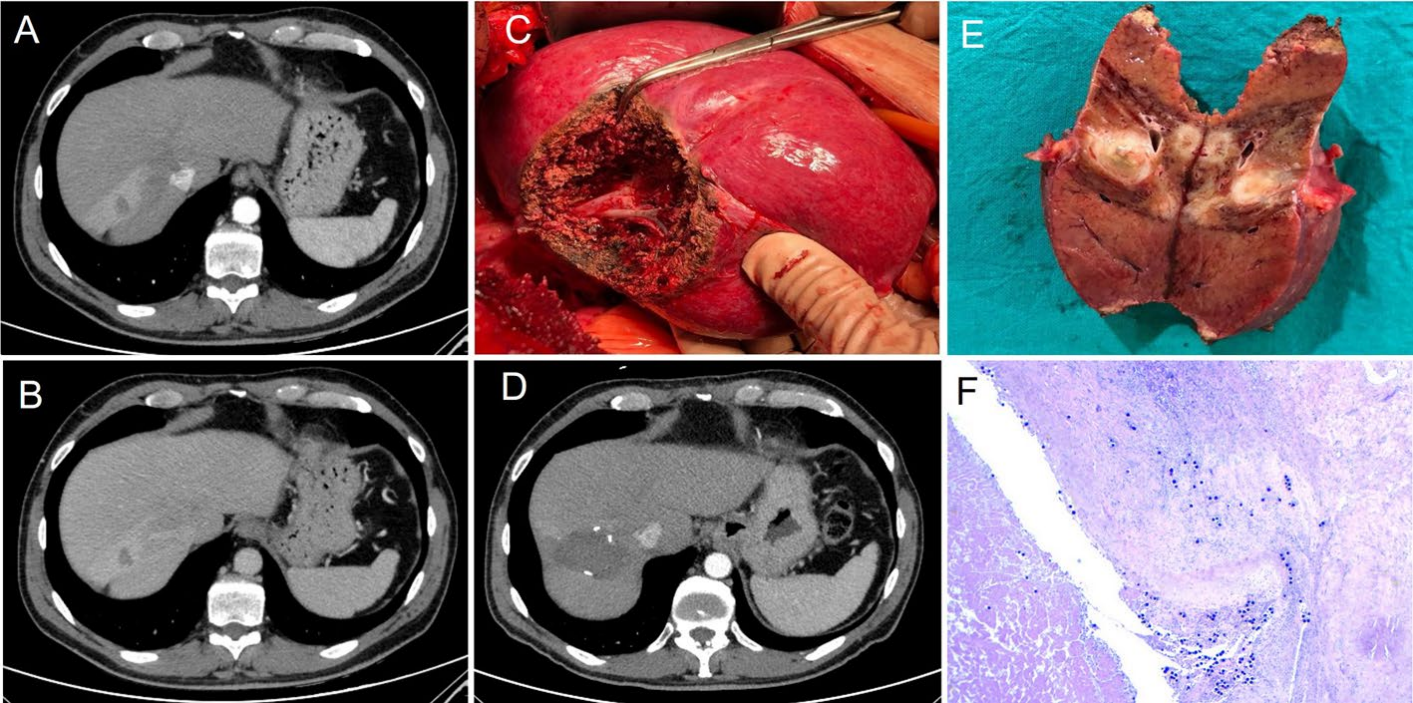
Post-treatment images of the patient diagnosed with HCC (Fig. 4): The CT study to assess response at 3 months (A and B) showed a 15-mm focal lesion in segment VII without contrast enhancement that could suggest tumor persistence or recurrence. After hepatic segmentectomy (C and D) performed 9 months after radioembolization, a resection cavity was shown, without focal hepatic lesions suggestive of tumor recurrence. Finally, the pathological study of the surgical piece (E and F) showed a fibronecrotic nodule in relation to the embolization material without the presence of residual neoplasia
